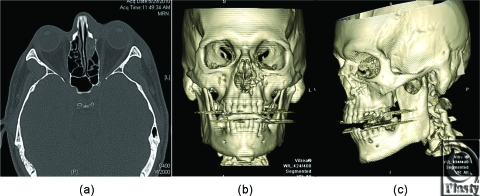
Postoperative maxillofacial computed tomographic scan with 3D reconstruction (a) axial view, (b) anteroposterior 3D view, (c) oblique 3D view.
